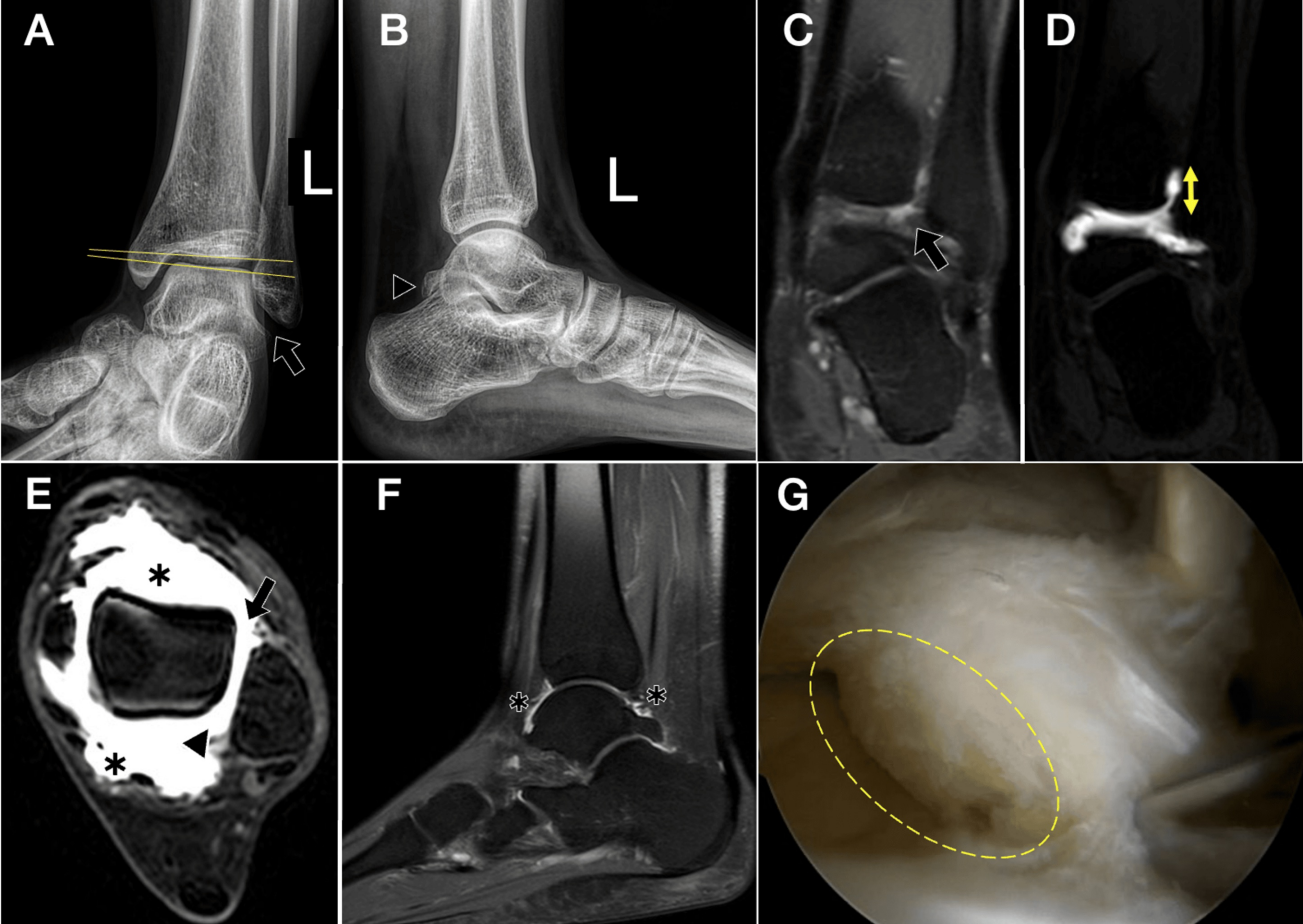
Imaging findings in anterolateral ankle instability in a 36-year-old female. (A) Anteroposterior (AP) radiograph showing loss of tibio-talar parallelism with widening of the lateral clear space (arrow). (B) Lateral radiograph demonstrating normal osseous alignment in this projection and a prominent Stieda process (black arrowhead). (C) Coronal STIR MRI at the level of the syndesmosis showing interposed edema within the distal tibiofibular syndesmosis (black arrow). (D) Coronal post-contrast T1 fat-suppressed MRI after intra-articular contrast (direct MR arthrography) demonstrating contrast ascending into the distal tibiofibular syndesmosis >7 mm above the joint line (double-headed yellow arrow). (E) Axial T1 spin-echo fat-suppressed image after intra-articular contrast: anterior and posterior talar recesses are distended with contrast (*). The anterior arrow indicates a full-thickness ATFL tear (absence of fibers); the arrowhead marks contrast at the level of the PTFL. (F) Sagittal pre-contrast STIR image through the tibiotalar joint showing enlarged anterior and posterior recesses (*) associated with a prominent Stieda process. (G) Arthroscopic image (posterior approach) in the same patient demonstrating the Stieda process with fibers of the PTFL and entrapment of the FHL tendon (yellow dashed ellipse). ATFL: anterior talofibular ligament; FHL: flexor hallucis longus; MR: magnetic resonance; PTFL: posterior talofibular ligament; STIR: short tau inversion recovery Images belong to the authors; patient consent was obtained.
